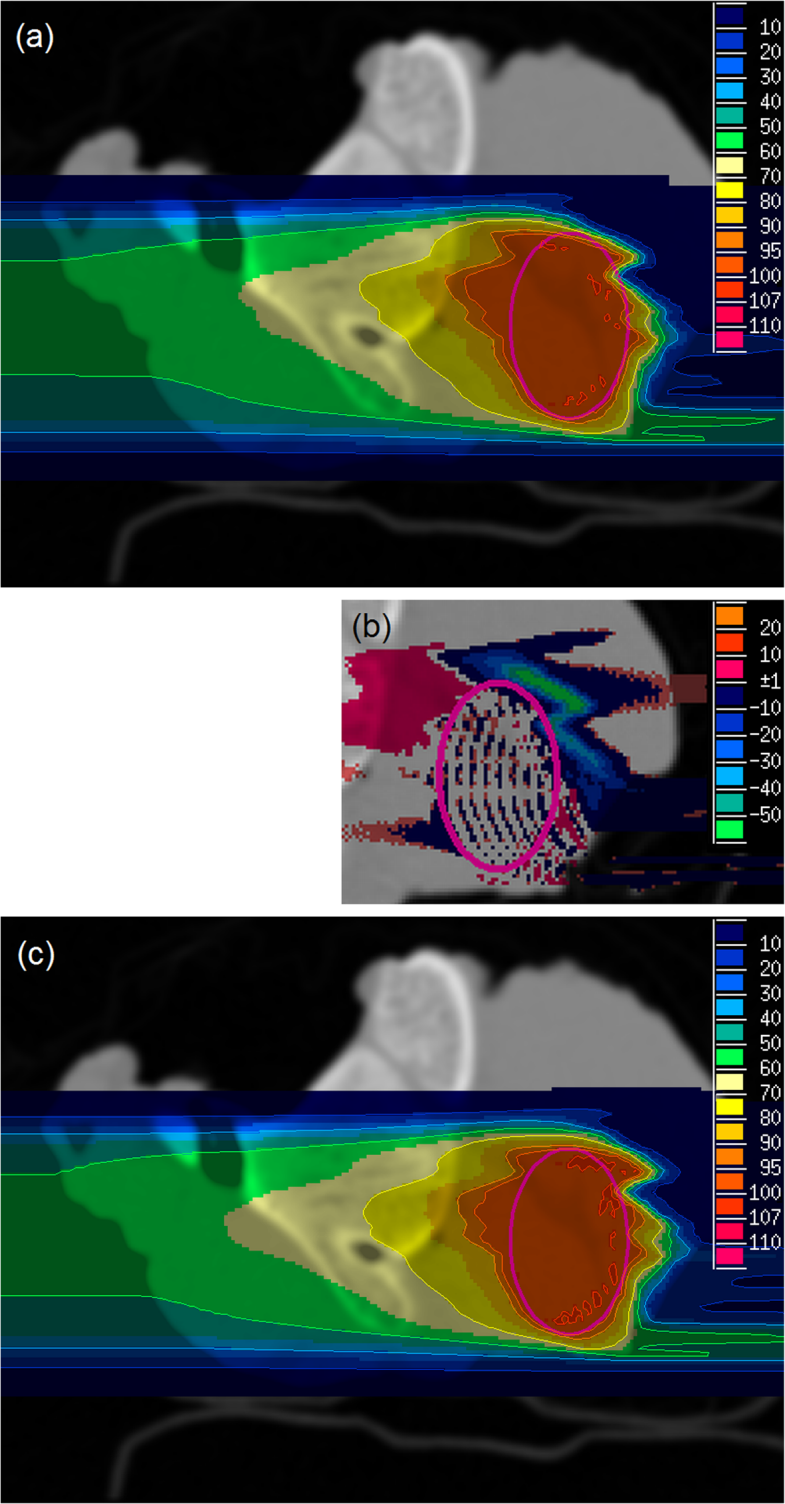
Carbon ion plans of sample 2.(a) optimized on p CT and recalculated on r CT; (b) dose difference of (a) and (c); (c) optimized and calculated on r CT. The PTV size was 17 × 26 × 16mm^3^ and the unit of color scale is percent of the prescribed physical dose.
